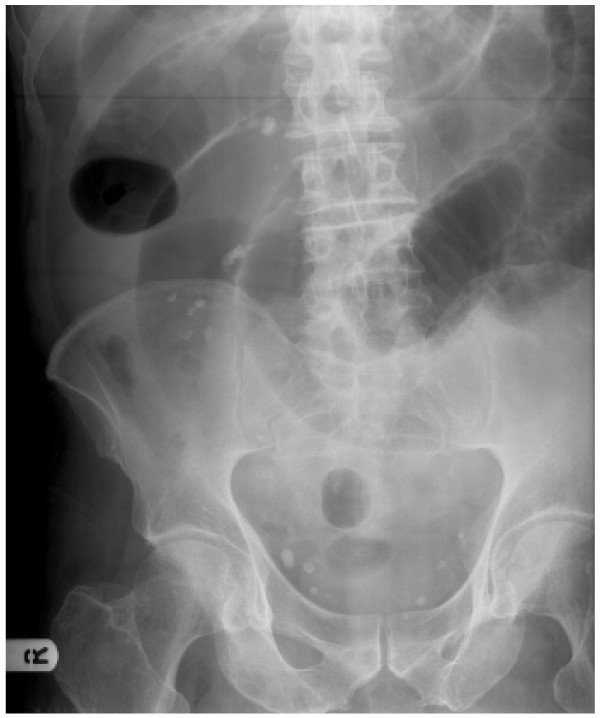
This x-ray shows multiple loops of dilated small bowel.
